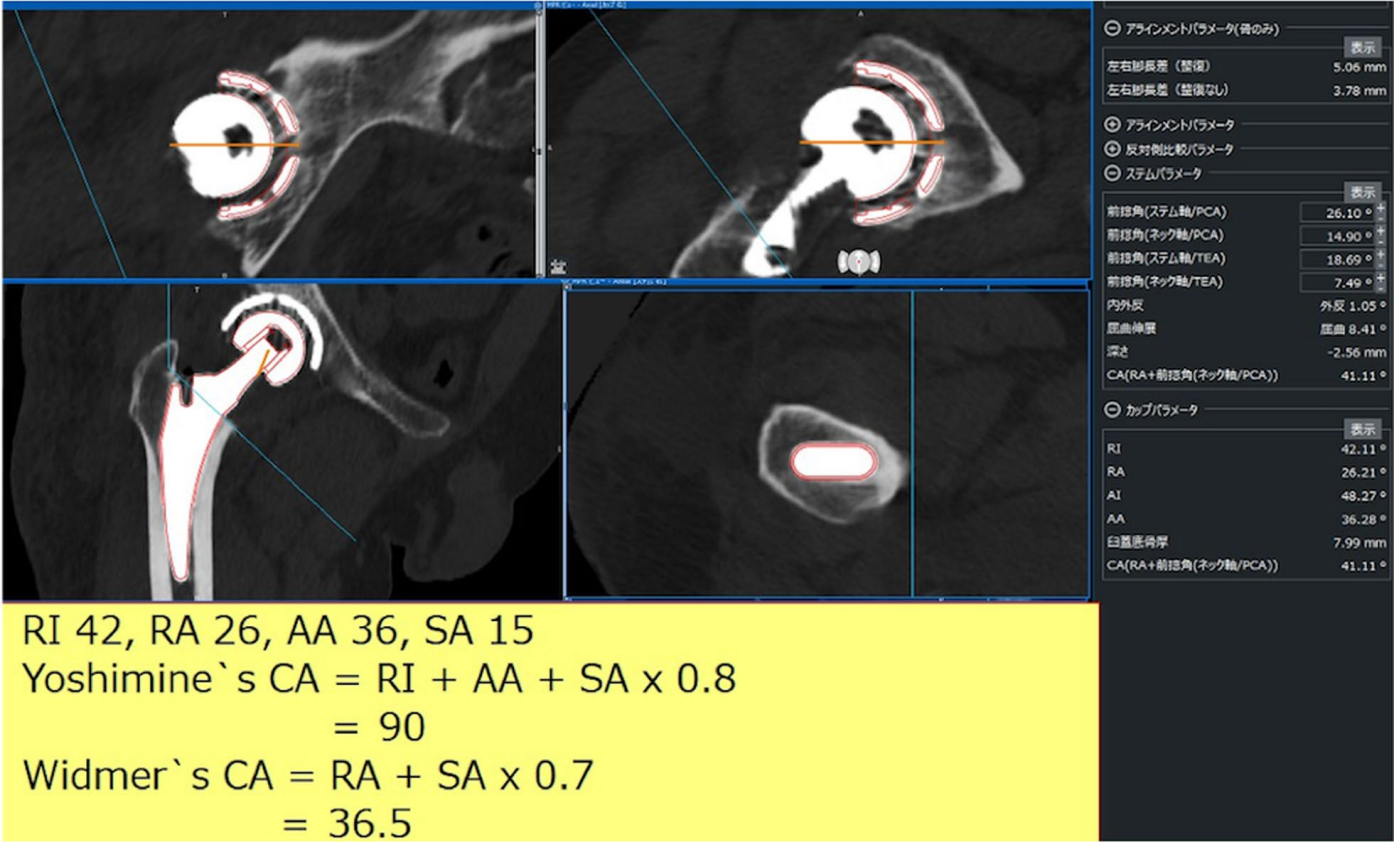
Implant orientations are measured by superimposing the CAD models of the implants on postoperative CT images using Zed Hip. Yoshimine’s CA and Widmer’s CA were calculated from the implant orientation measurements. CAD, computer-aided design; *CA* combined anteversion
